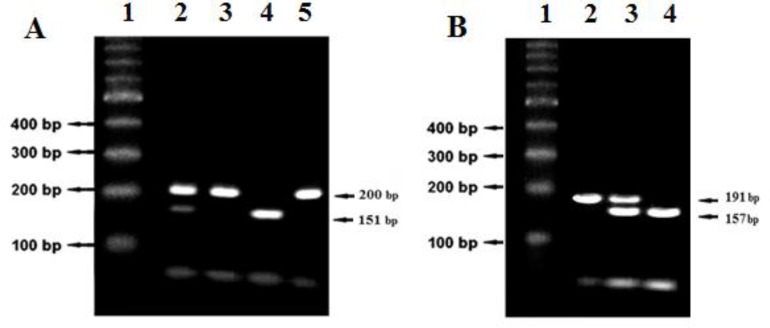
Genotyping of the *NQO1* C609T (A) and *CAT* C-262T (B) polymorphisms by *HinfI* and *EcoRV* RFLP. A) From left to right the lanes are 1) DNA size marker (100 bp ladder), 2) CT genotype (200, 151 and 49 bp), 3) CC genotype (200 bp), 4) TT genotype (151 and 49 bp) and 5. intact (200 bp) B) From left to right the lanes are 1) DNA size marker (100 bp ladder), 2) TT genotype (191 bp), 3) TC genotype (191, 157 and 34 bp) and 4) CC genotype (157 and 34 bp
